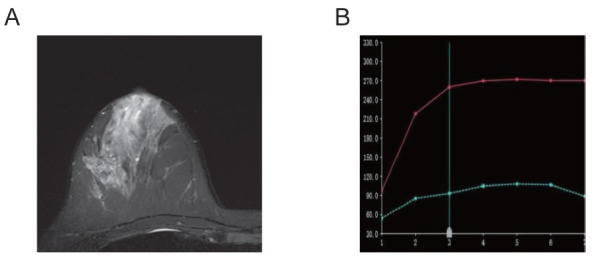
Right breast ductal carcinoma *in situ* (Luminal B subtype, HER2-negative). The lesion presents as a mass with heterogeneous, high signal intensity on T2-weighted imaging (A). The TIC demonstrates slow-to-moderate early enhancement (B).
